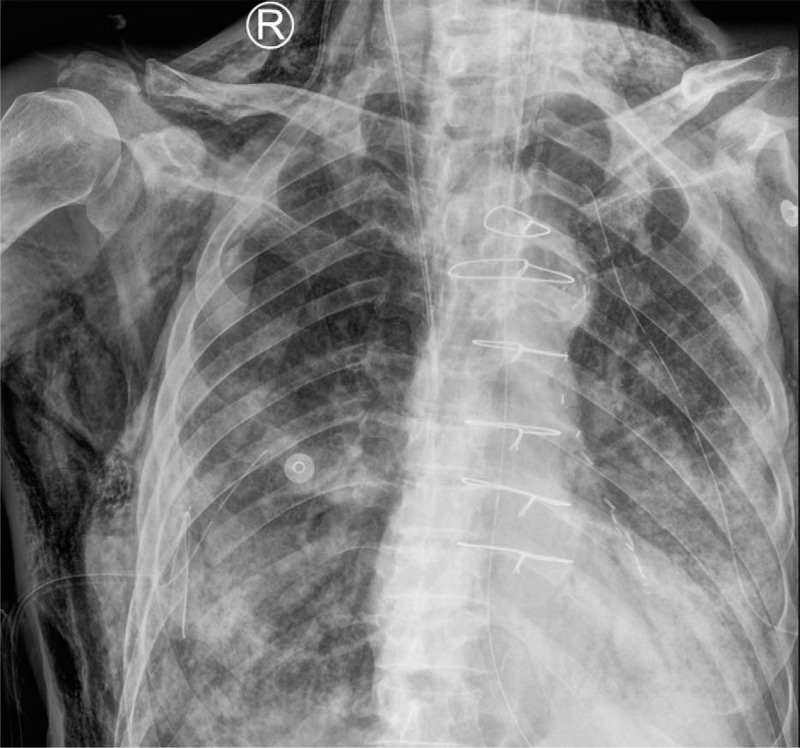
A 67-yr-old male resident of Wuhan with chronic cardiac and pulmonary diseases had dyspnea, accompanied by fatigue, and mild diarrhea. RT-PCR result was positive for SARS-CoV-2. He had a history of coronary artery bypass. He underwent invasive ventilation for correcting hypoxemia. Mobile X-ray was used for assessing SARS-CoV-2 infection instead of CT. The chest radiograph revealed extensive air-space opacities in bilateral lungs, with lower lung involvement more serious than upper lung. Extensive subcutaneous emphysema in neck and chest wall could be easily seen. Mediastinal emphysema and a small amount of pneumothorax were also identified, as well as enlargement of the left ventricle. The patients died of respiratory and heart failure 2 d after this scan.
